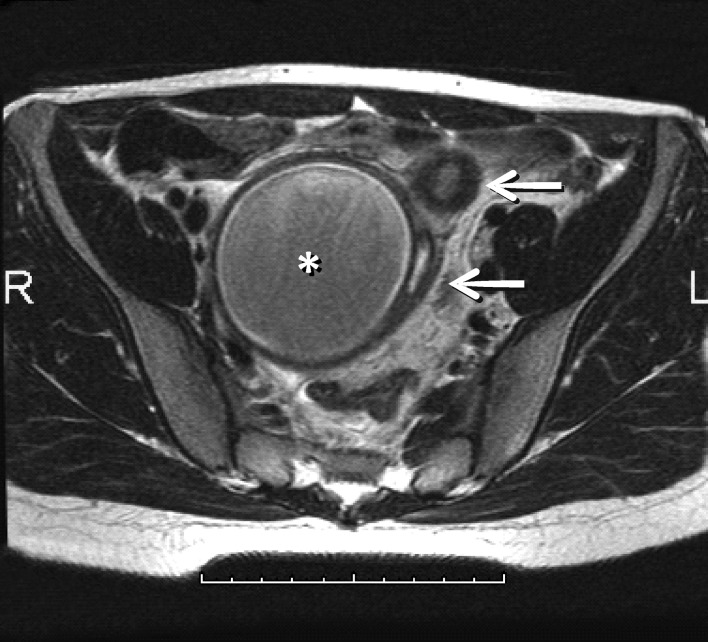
Figure 1:MRI showing dilated cavity in continuity with hydrosalpinx (arrows)
